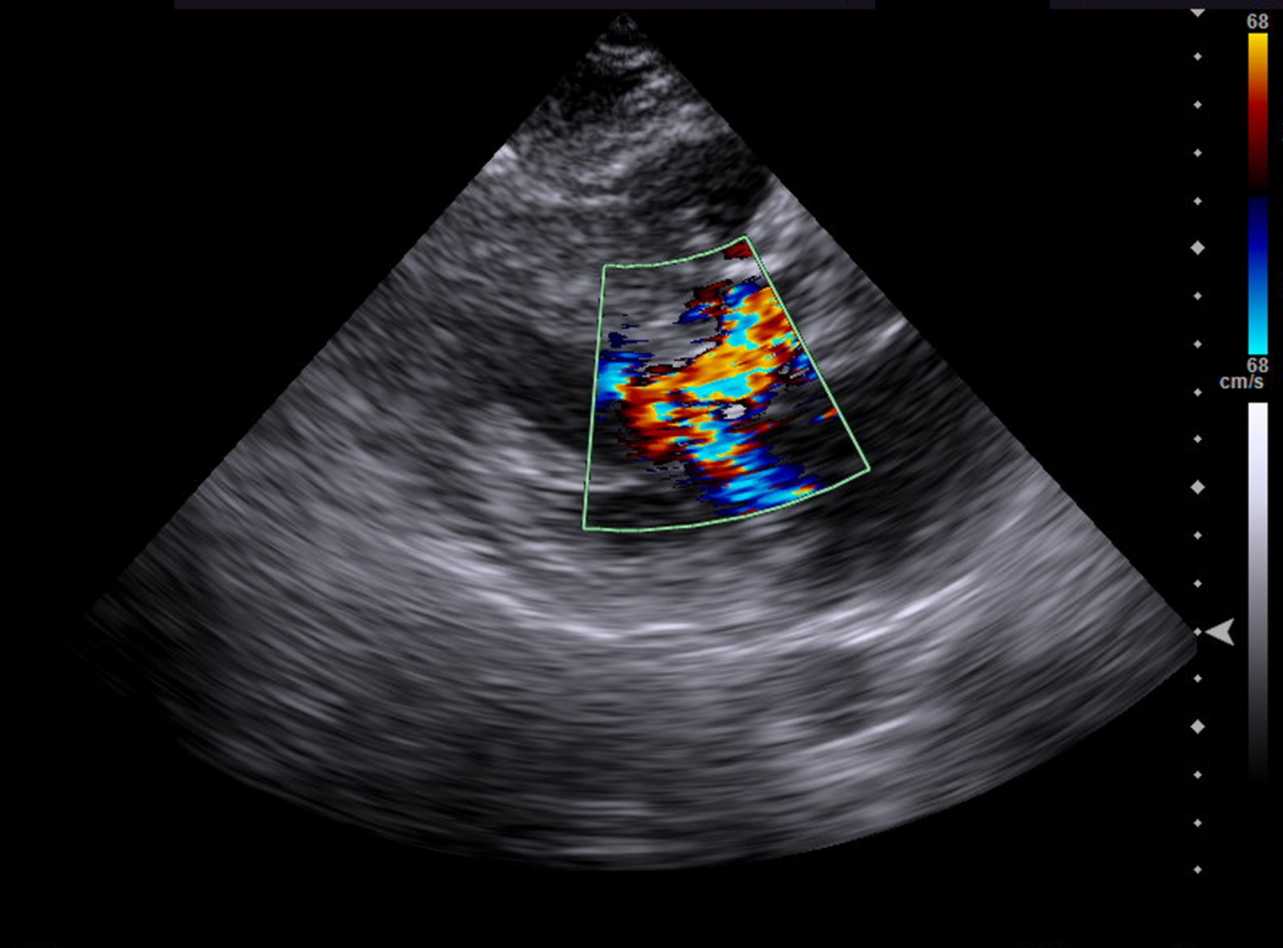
Color Doppler echocardiography Color Doppler echocardiography (systolic frame) shows marked turbulence in the left ventricular outflow tract (LVOT) and a posteriorly directed jet of mitral regurgitation. LVOT during systole is due to systolic anterior motion (SAM) of the mitral valve, resulting in a Venturi effect.
